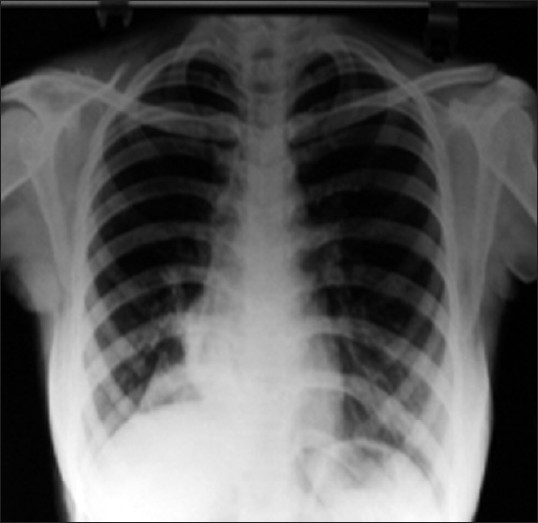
Chest X-ray (PA view) showing segmental collapse of right lower lobe
